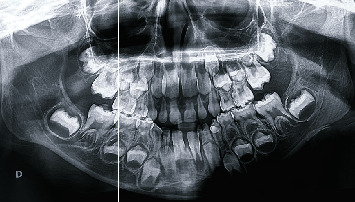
Panoramic radiograph showing the absence of dental abnormalities in the permanent teeth.
